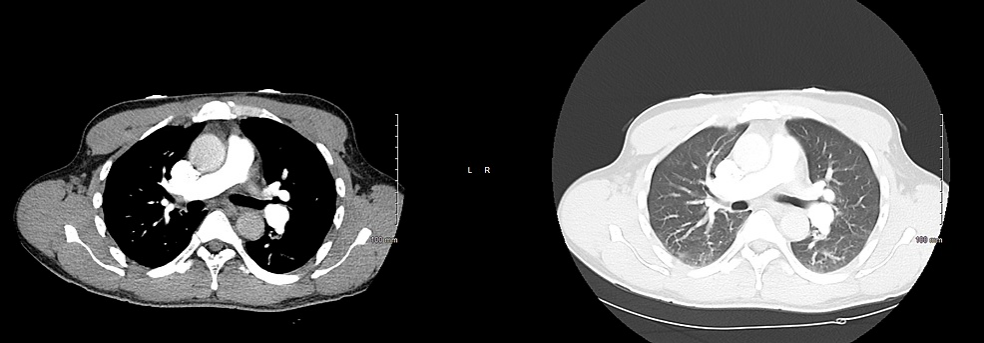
CT angiogram of the chest with no findings suggestive of pulmonary embolism
